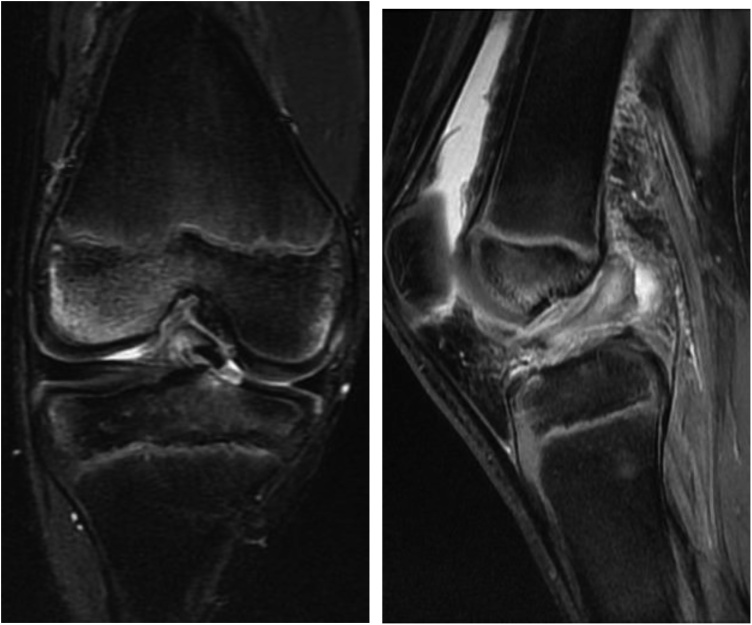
Magnetic resonance imaging revealed an osteochondral fragment in the intercondylar fossa and a signal change in the anterior cruciate ligament midsubstance.
